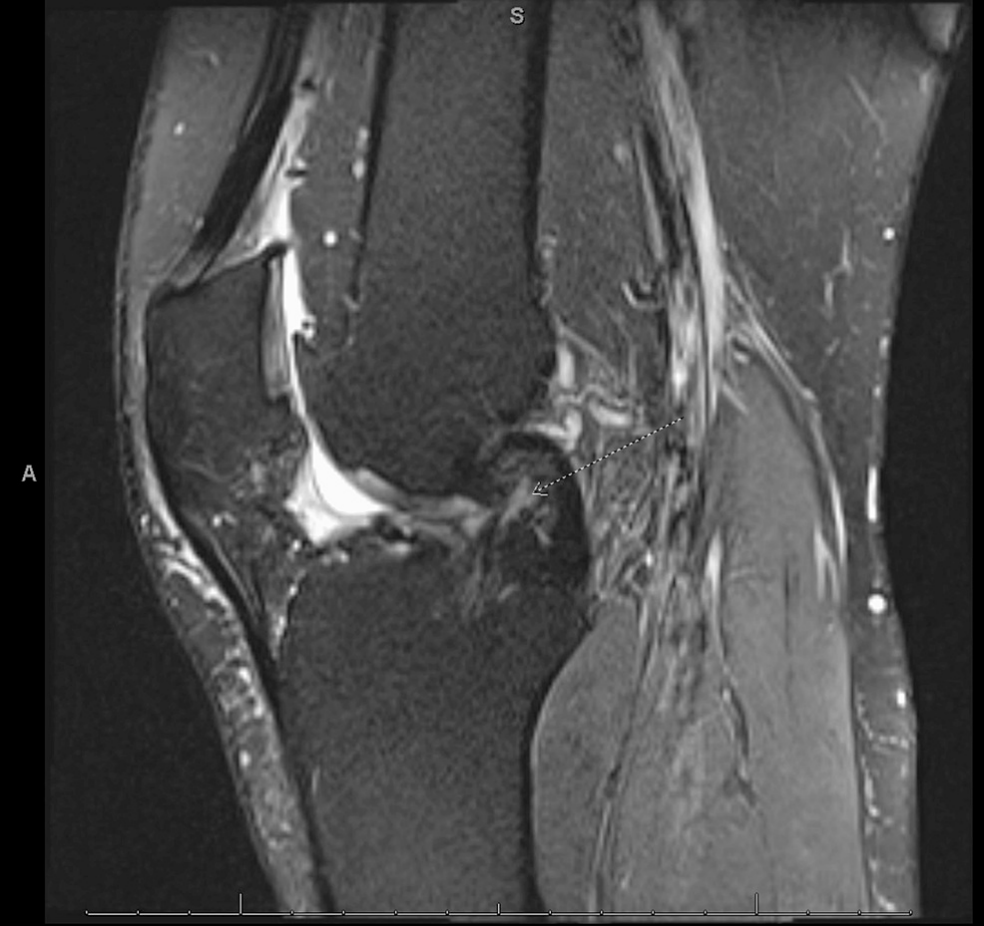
MRI of right knee, lateral view showing previous ACL graft (arrow) with signal changes concerning for a graft tear. ACL: anterior cruciate ligament
